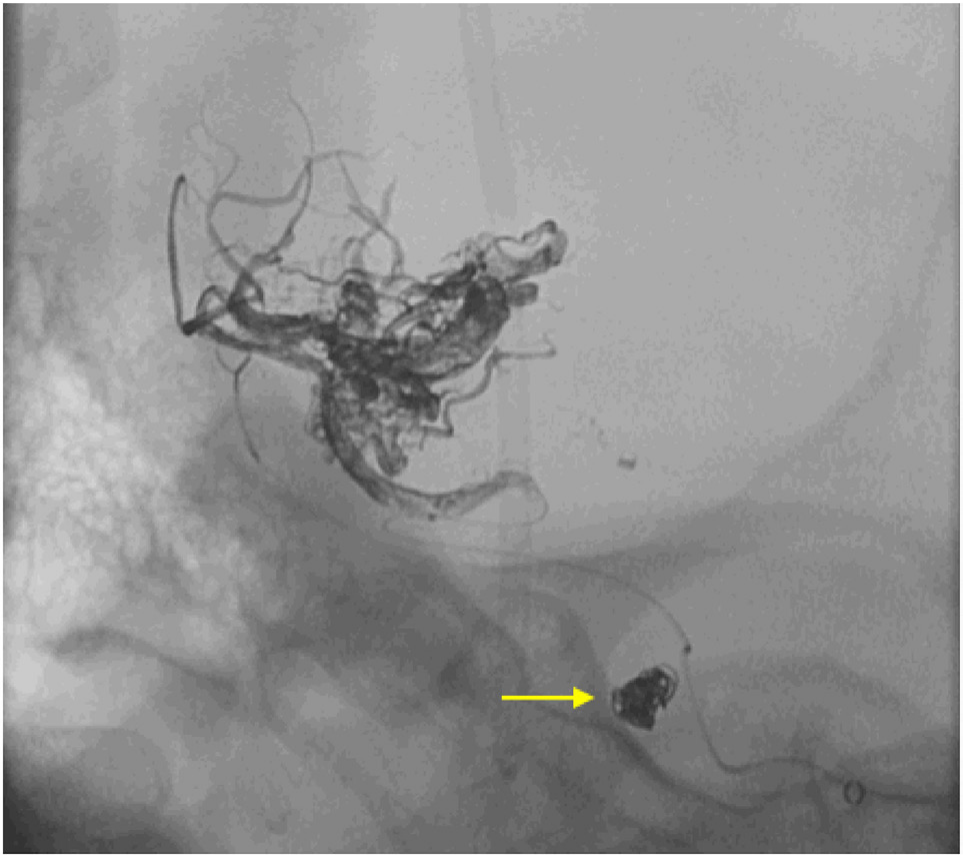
Final run X-Ray demonstrating the position and configuration of the platinum coils inside the sac of the aneurysm (yellow arrow) as well as the ONYX filling the vascular channels of the arteriovenous malformation after the procedure.
